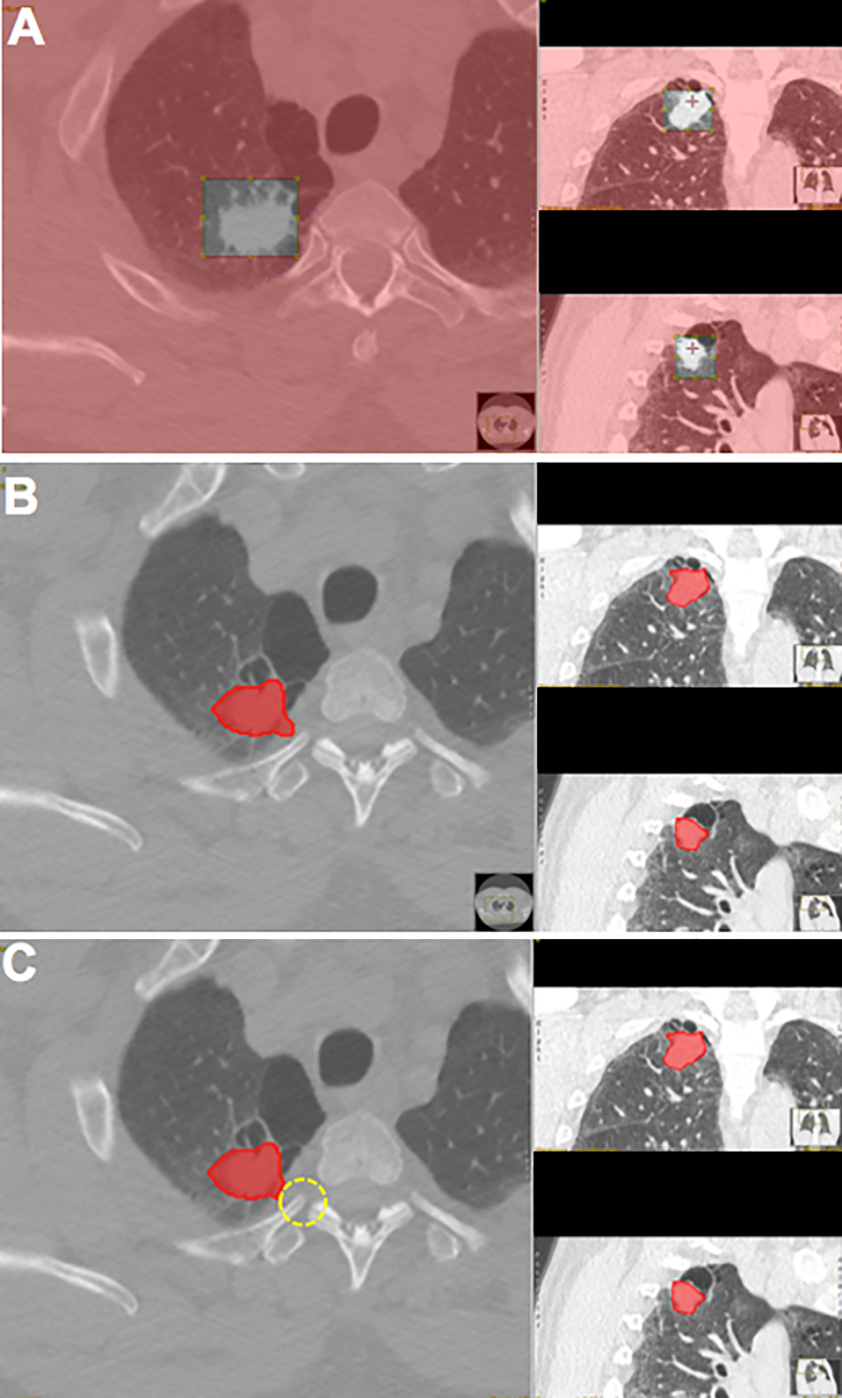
CANARY segmentation process. CT scans from a cohort of biopsy-confirmed ADCs from VUMC/TVHS (n = 50) and Mayo (n = 45) were analyzed by three independent software users from two institutions using CANARY segmentation. All ADCs were <3 cm, according to previous CANARY studies. A pulmonologist and radiologist confirmed nodule location prior to segmentation. A. Once the observer had identified the nodule on the CT scan, placing the pointer over the nodule established a volume of interest around the nodule. B. Next, CANARY defined the nodule border (red area) on each CT slice. C. If the border appeared inconsistent with the perceived nodule edge (i.e. extension into vasculature or chest wall), the observer adjusted the nodule borders by using an eraser tool (dotted yellow circle).
